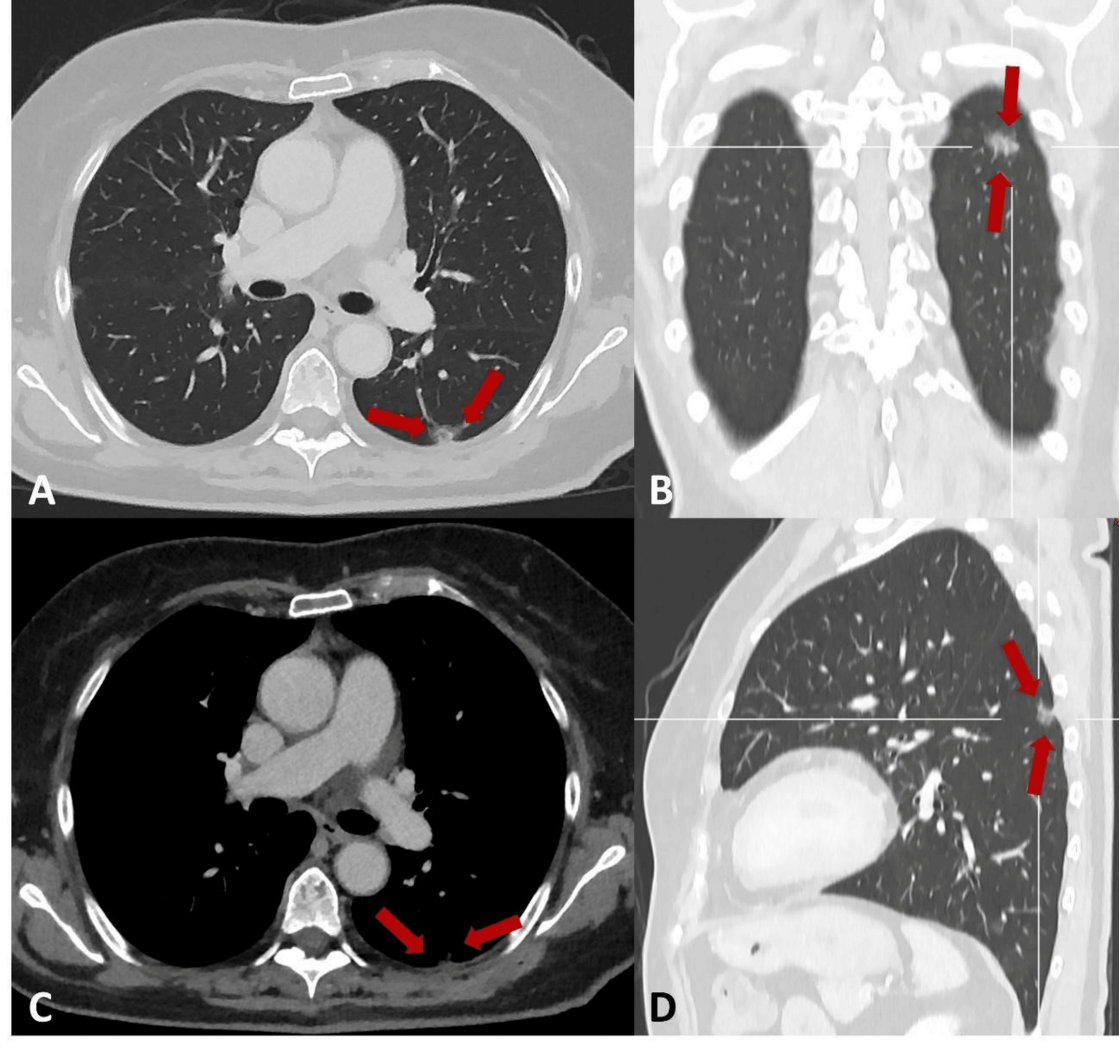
Chest CT showing a subsolid area in the upper segment of the left lower lobe (red arrow), predominantly ground-glass and ill-defined. (A) Lung window axial plane. (B) Lung window coronal plane. (C) Soft tissue window with contrast axial plane. (D) Lung window sagittal plane. CT: computed tomography
